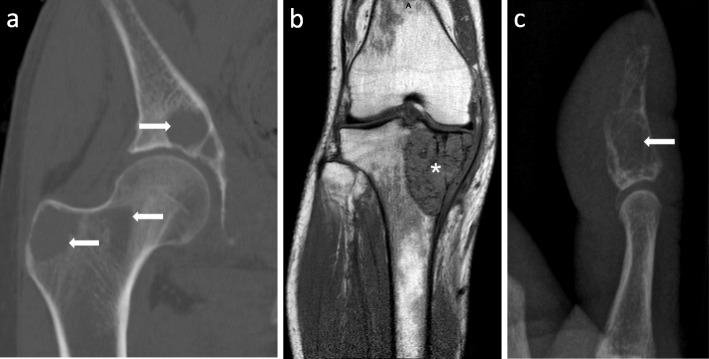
Imaging studies of selected osteolytic lesions. a. CT scan of the right hip. Osteolytic lesions are present in the acetabulum, femoral neck and trochanter majus (arrows). b. MRI scan showing a large brown tumor in the head of the right tibia (*). c. x-ray of the left thumb reveals osteolytic destruction of the end phalanx (arrow)
